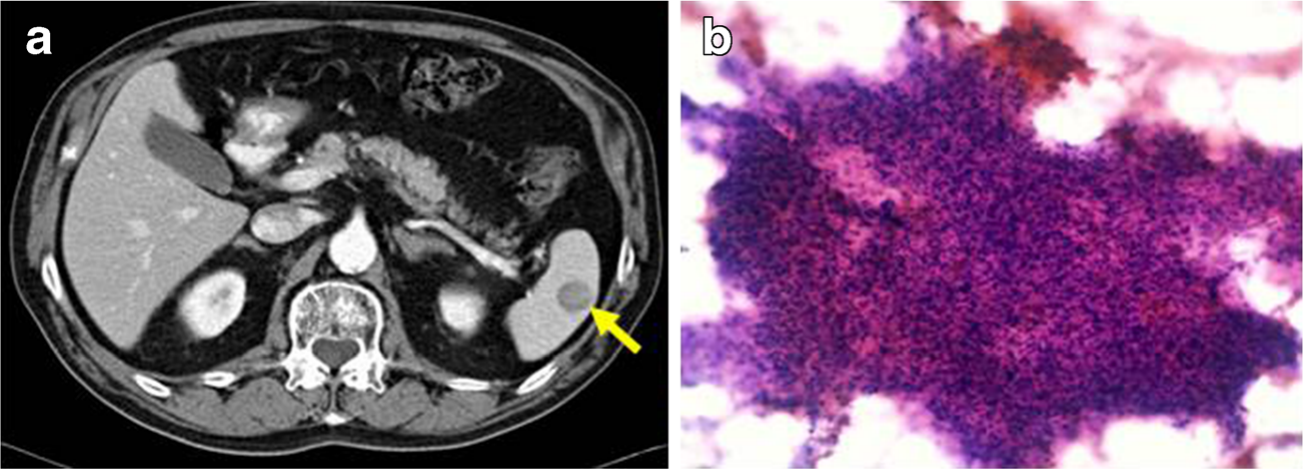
PATHOLOGY: IMT of the spleen. a Axial reformation of contrast-enhanced CT on portal phase. Solid and well-defined lesion, hypodense to the surrounding spleen parenchyma. b Sample obtained with fine-needle aspiration. Prominent cellularity composed of an irregular, weakly basophil set of mioepithelial-fusiform cells. Numerous inflammatory cells (plasmatic, lymphocytes and few neutrophils and eosinophils) with isolated macrophages were also found. No atypia or mitotic figures were present
